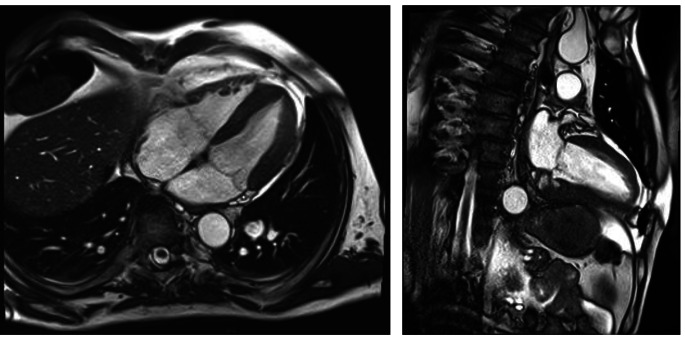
Intramyocardial abscess seen at cardiac MRI
